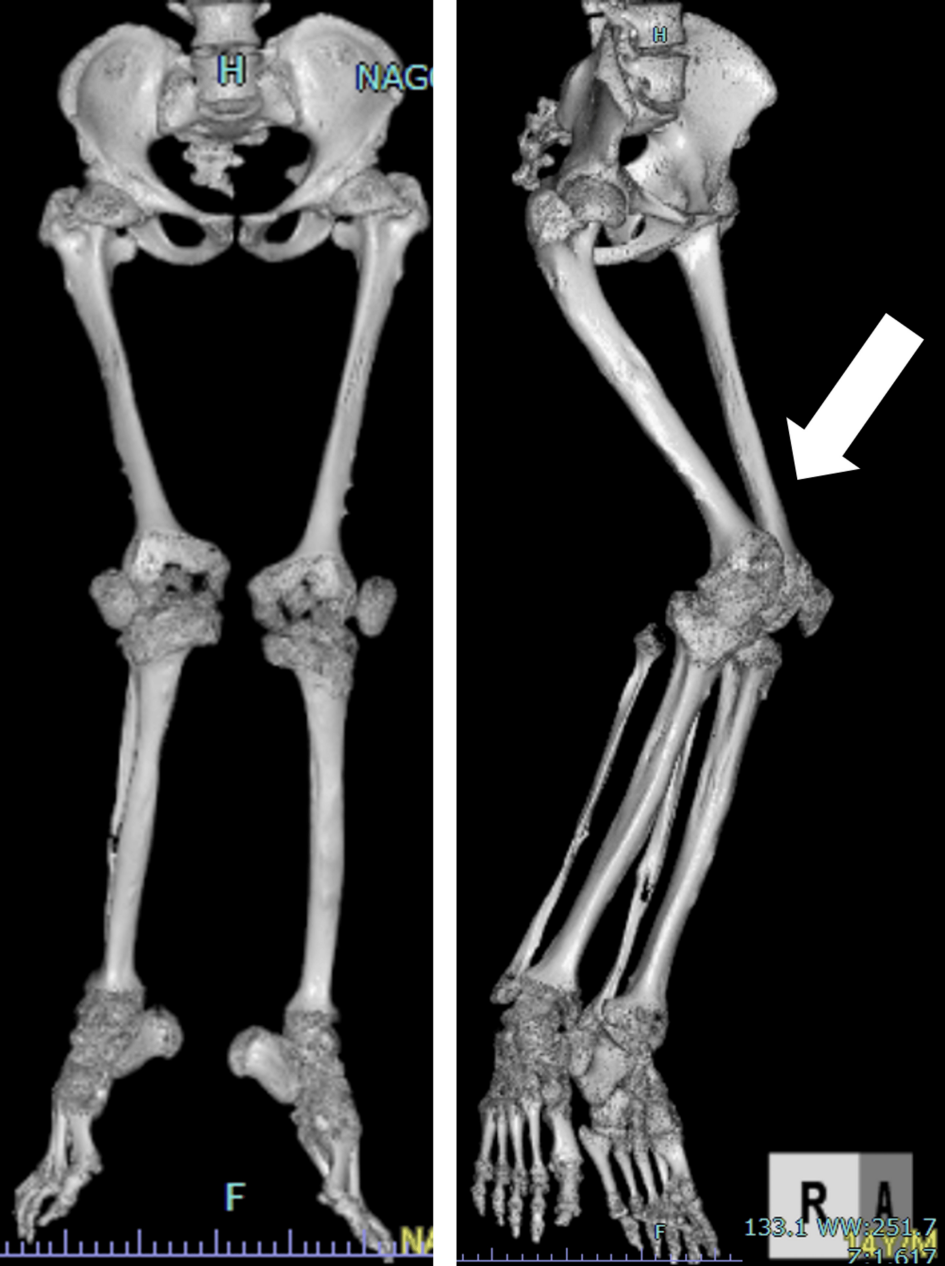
Preoperative CT images As pointed to by the white arrow, knee flexion contracture was observed in addition to valgus knee deformity and permanent patellar dislocation.
